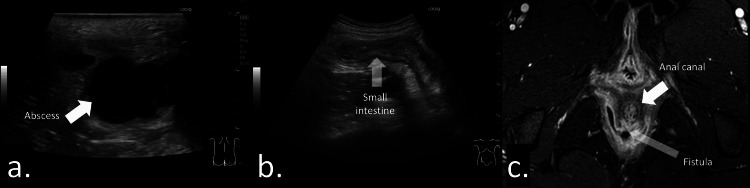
Inflammatory disease presenting with anal pain at the 12 o’clock position in a 15-year-old female. (a) Sonographic image demonstrating a large perianal abscess. The fistulous tract originating from the anal crypt could not be visualized because of the large lesion size. (b) Abdominal sonogram showing inflammatory changes in the terminal ileum. (c) Axial gadolinium-enhanced, fat-suppressed T1-weighted magnetic resonance image obtained after surgical incision, demonstrating a fistulous tract arising from the anal canal at the 6 o’clock position.
